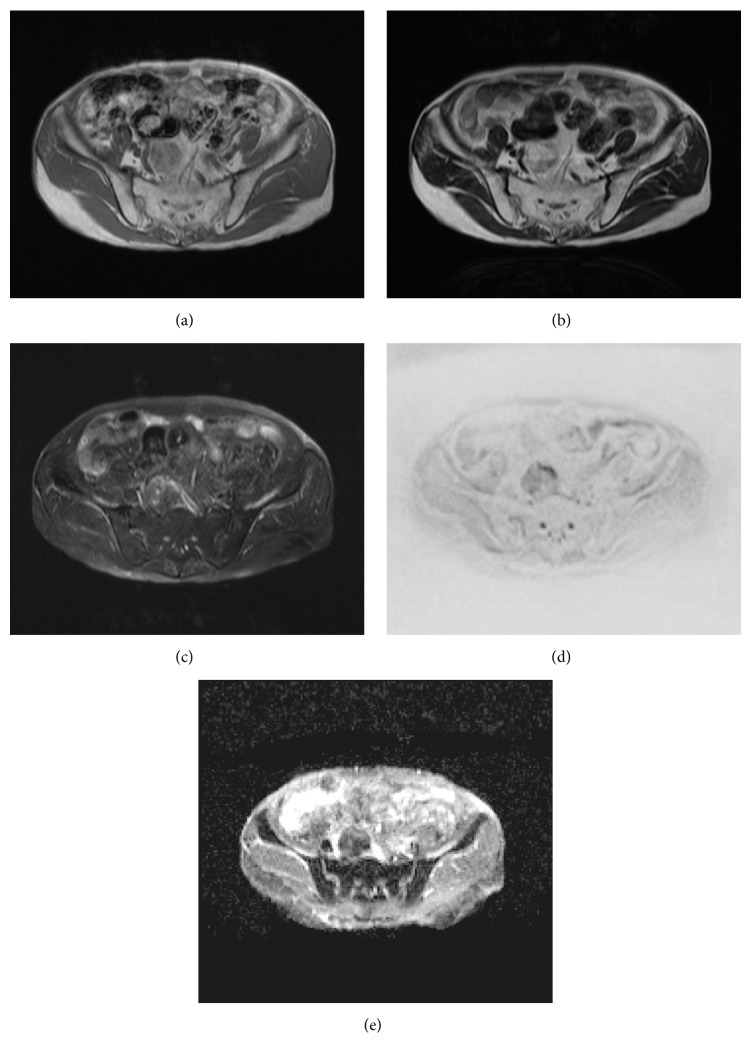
MR images showed a round heterogenous tumor in the presacral space ((a) T1-weighted, (b) T2-weighted, (c) fatty-saturated T2-weighted, and (d) diffusion-weighted image (*b* = 800) and (e) ADC map).
